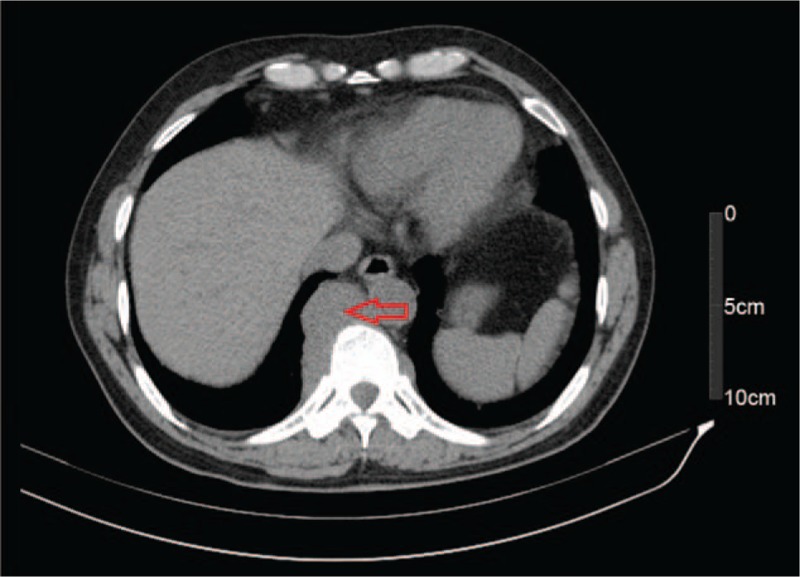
Computed tomography revealed a space-occupying lesion on the right side of centrums 9–11 of the thoracic vertebrae (red arrow).
